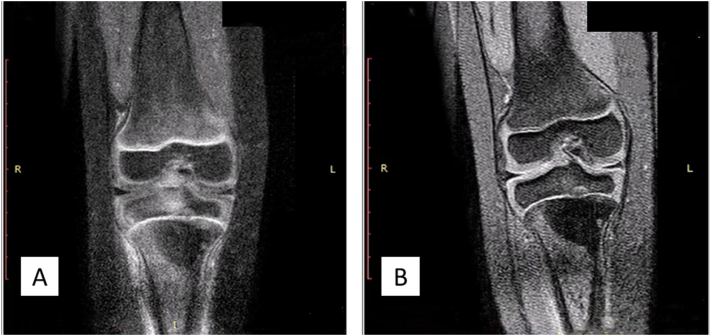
MRI examination of the right proximal tibia. A) T1 coronal MRI examination; B) T2 coronal MRI examination.
